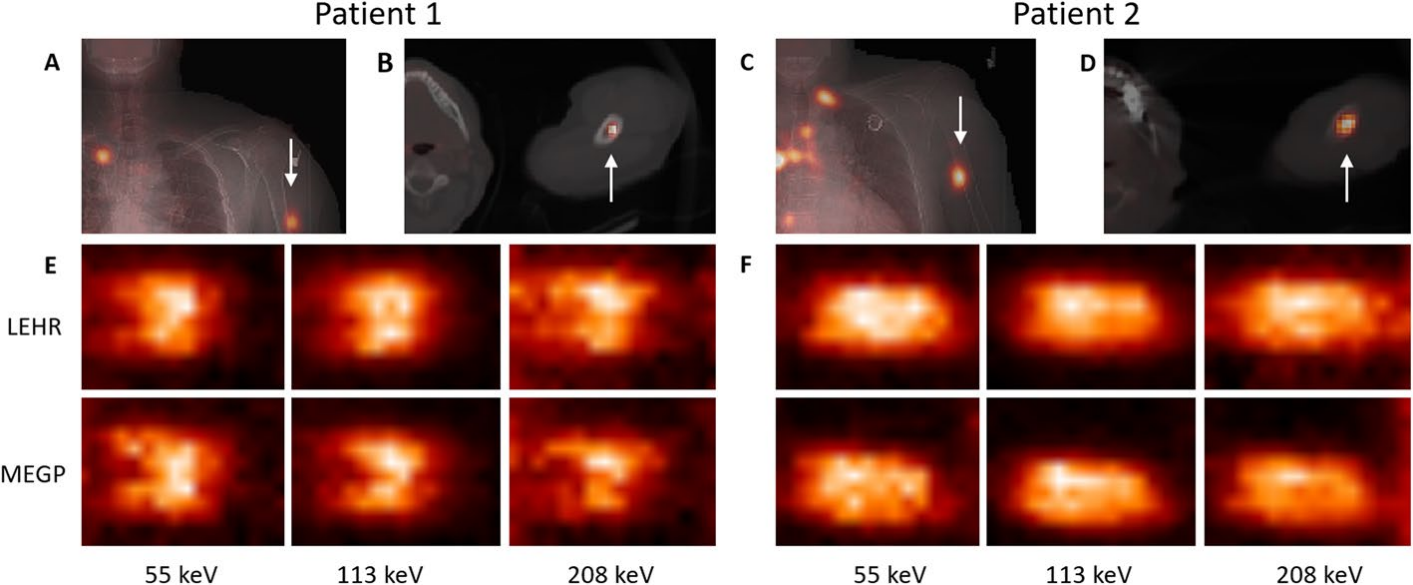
Example images for patient 1 (left) and patient 2 (right). Top row are A, C conventional gamma-camera images overlaid on the CT localizer, and B, D [^68^Ga]Ga-DOTA-TATE PET/CT images for tumour localization. Bottom panels show images from the hand-held camera acquired at day 1 with the LEHR and MEGP collimators, in the three energy windows. Images have been cropped for better visualization. Note that the orientation of the hand-held camera images depends on the camera positioning and does not correspond to that of the conventional camera systems. Panels A and E are adapted (cropped and re-arranged from material released under a Creative Commons Attribution 4.0 International License, https://creativecommons.org/licenses/by/4.0/) from [11]
